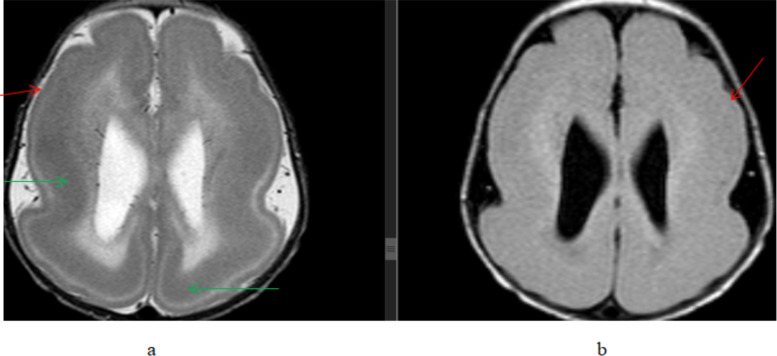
An 8-month-old female patient presented with developmental delay and seizure, (a) T2W axial and (b) FLAIR axial, brain MR images showing bilateral cerebral hemisphere smooth surface outline(red arrows) with a subcortical band of T2 hypointensity(green arrow). Findings are consistent with lissencephaly with band heterotopia
